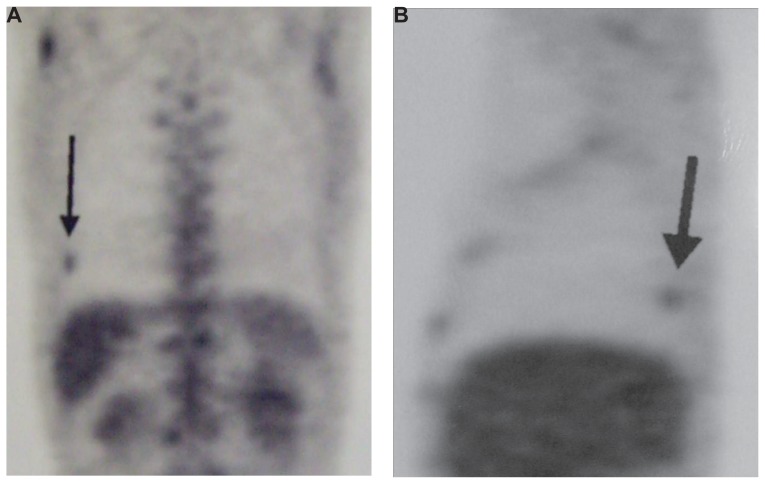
PET scan demonstrating a focus of intense FDG activity (arrows) in the right lung basis.
